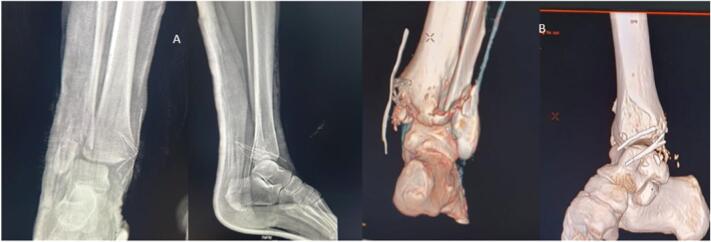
An X-ray image of the ankle after reduction with missing medial malleolus B: 3D image after the CT scan.
